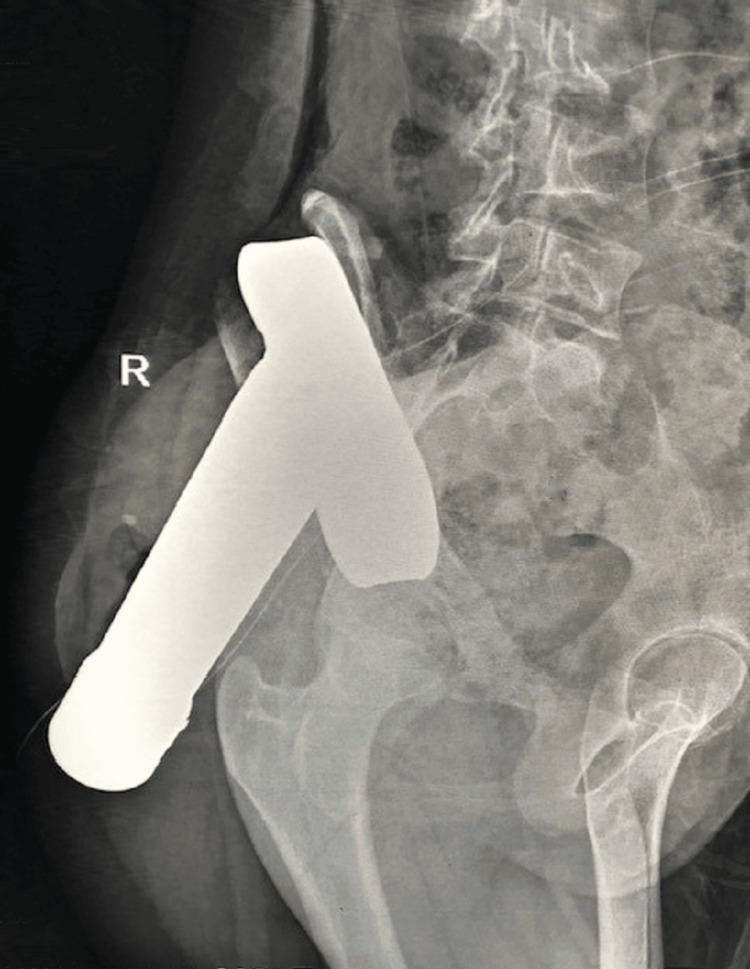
X-ray scan of the abdomen and pelvis demonstrating the trajectory of the metallic foreign body. The rod is seen passing through the right gluteal region and penetrating the right iliac blade without breaching the sacroiliac joint or acetabulum.
